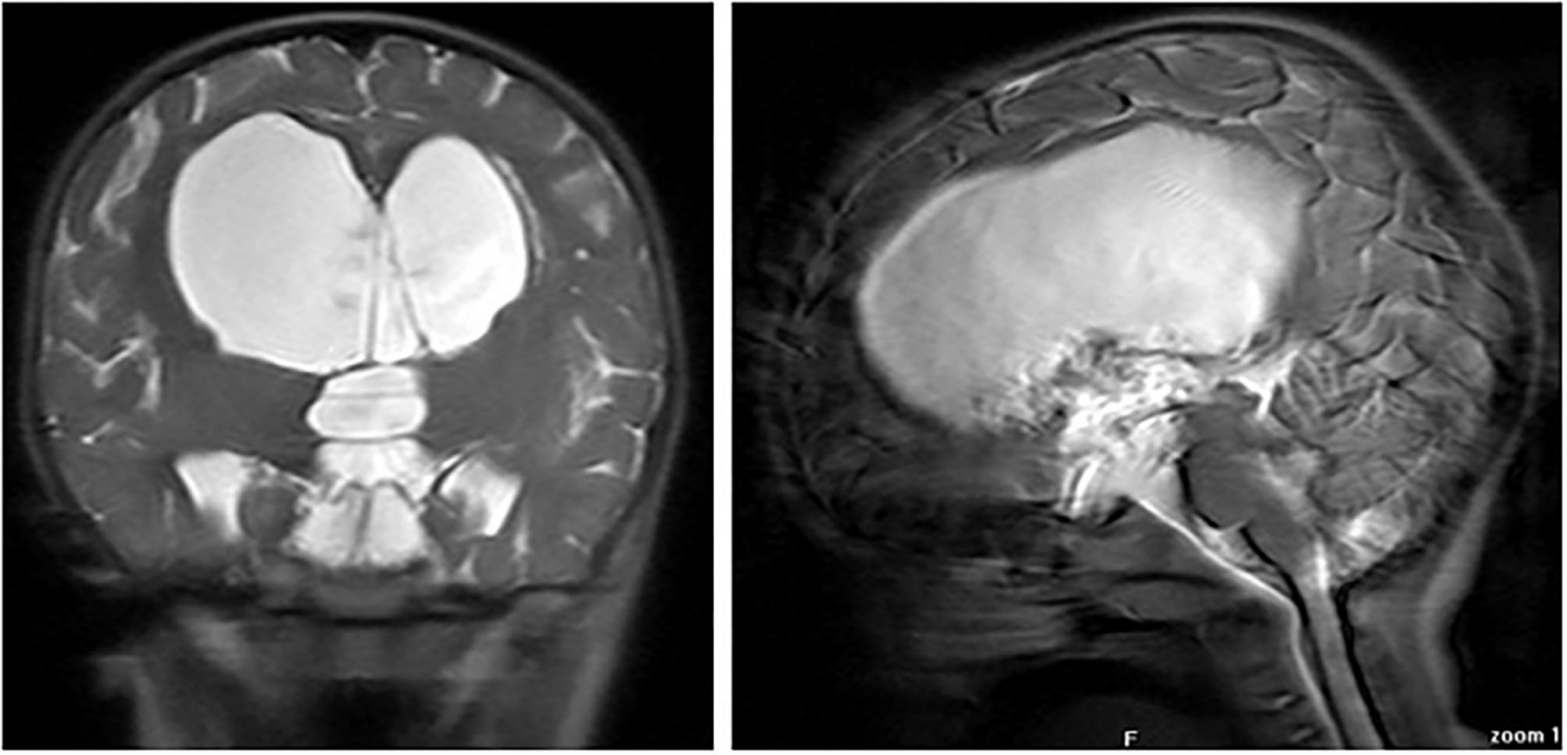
Coronal (right) and sagittal (left) MR images at diagnosis, showing large suprasellar arachnoid cyst.
